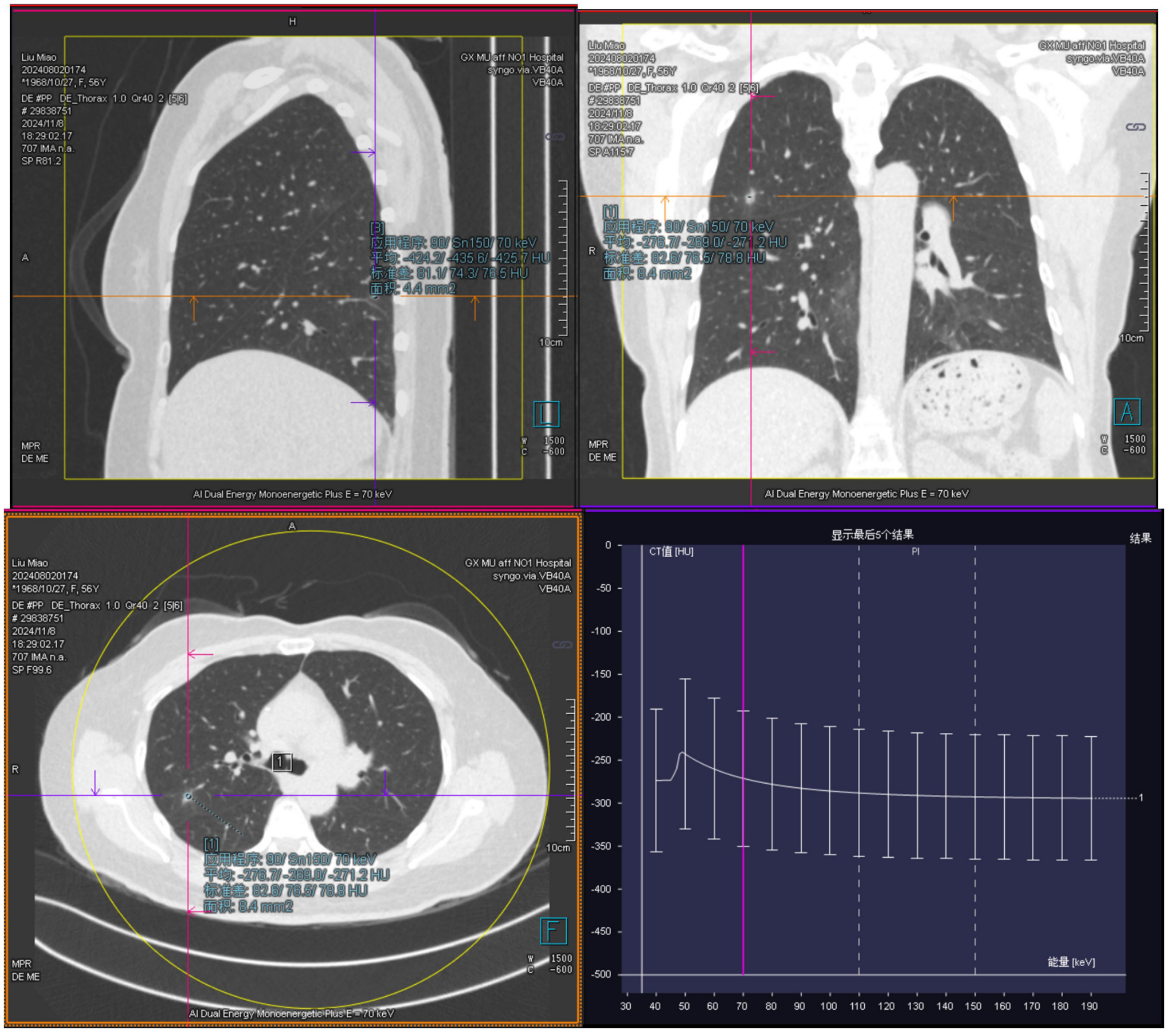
Ground-glass nodules are visible in the lungs on chest computed tomography (CT), with a close relationship to the surrounding blood vessels.
